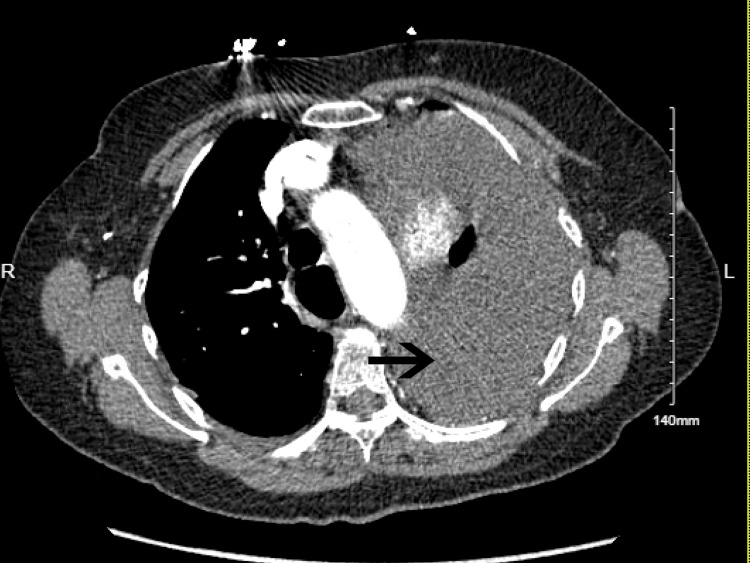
CT angiography of the revealing large left-sided hydropneumothorax.
